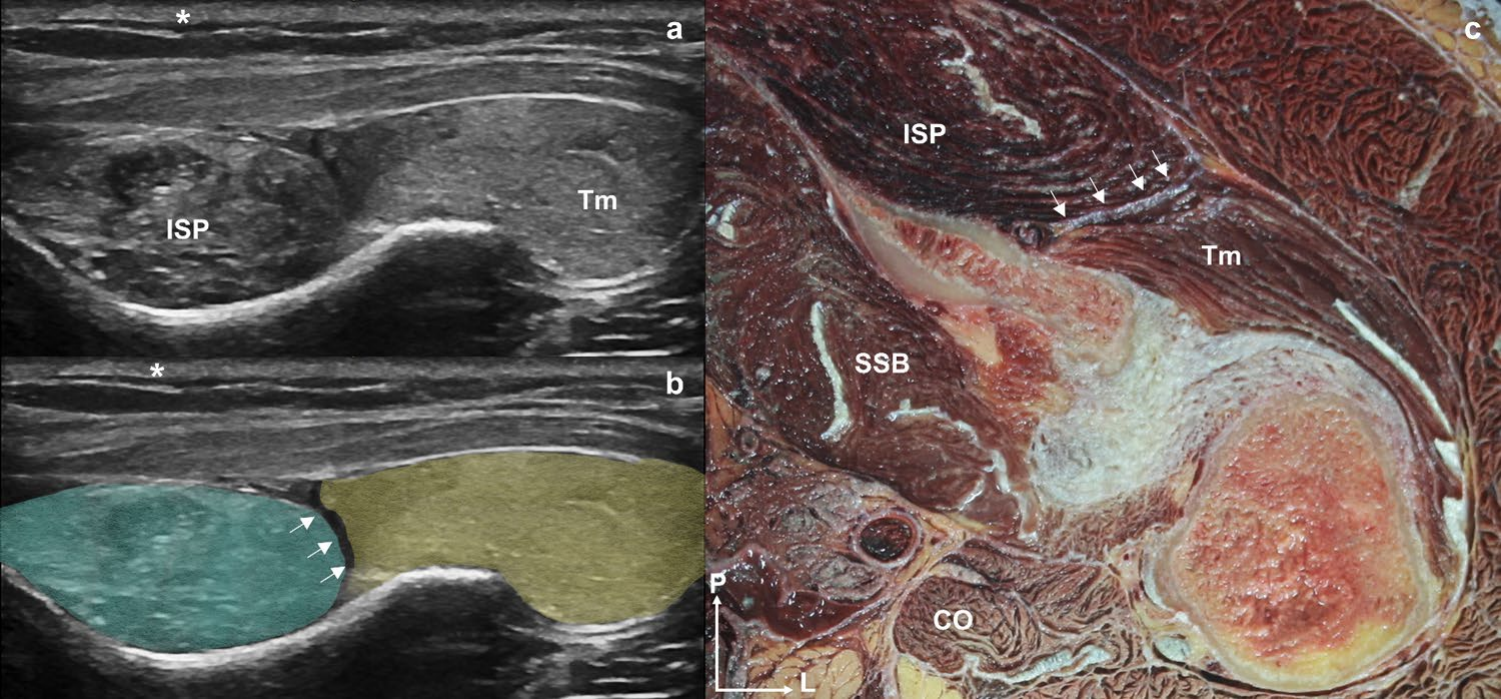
Images a and b are the same ultrasound cross-sectional view of the left shoulder that shows the fascial septum (white arrows) separating the infraspinatus muscle from the teres minor. b The infraspinatus is highlighted in blue, and the teres minor in yellow. The skin is marked with an asterisk (*). c Superior view of a transverse anatomical section of the left shoulder joint showing the infraspinatus and teres minor muscles and the fascial septum that separates them (white arrows). Abbreviations *ISP*: infraspinatus, *Tm*: teres minor, *CO*: coracobrachialis
